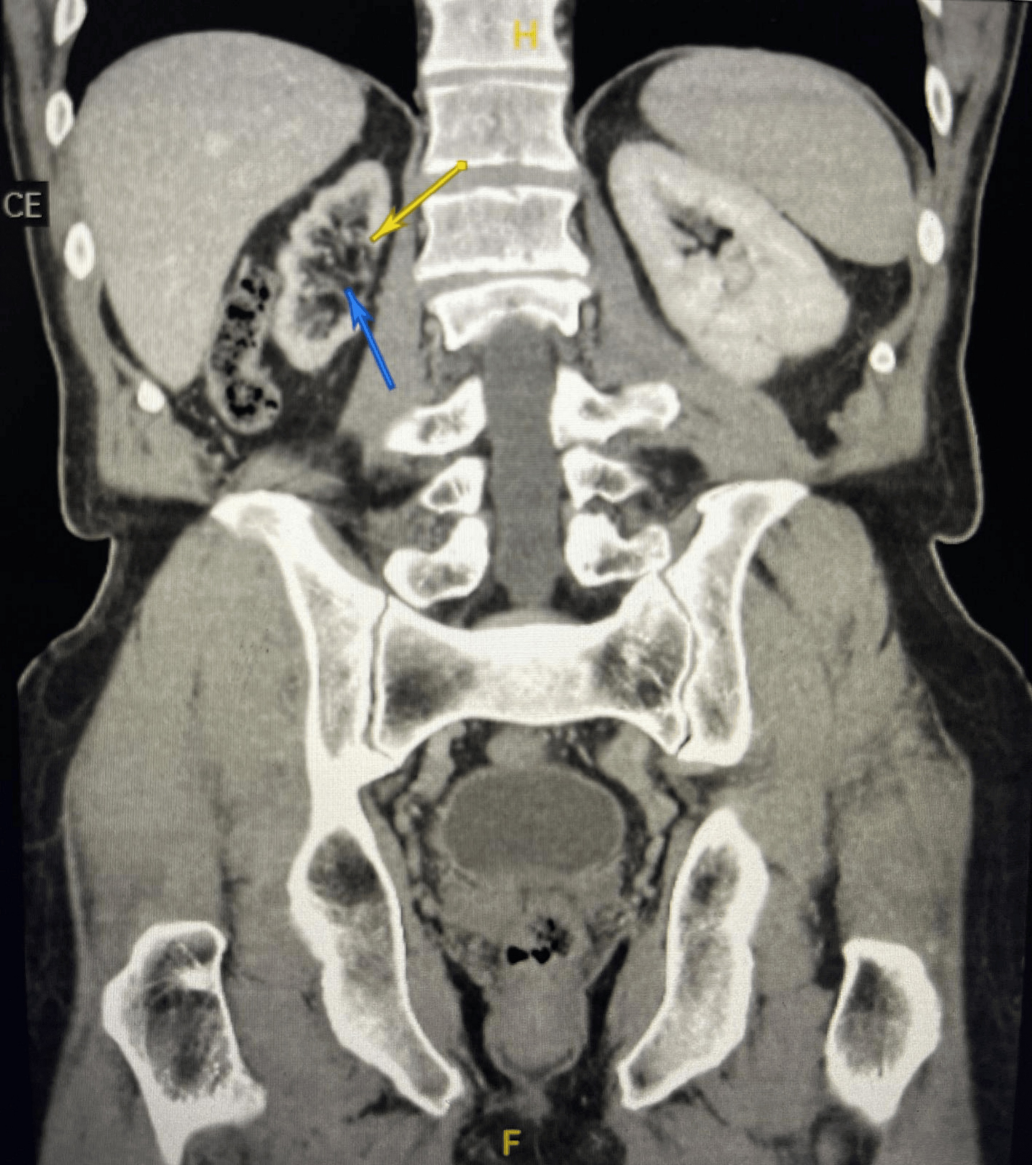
Follow-up CT showing resolution of the staghorn calculus Follow-up contrast-enhanced computed tomography scan obtained approximately one year later demonstrating complete radiological disappearance of the previously identified right staghorn calculus, with persistent right renal atrophy.
